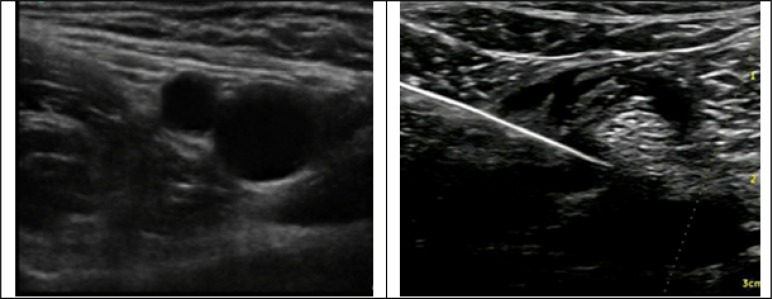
Ultrasonography view of right inguinal structures
